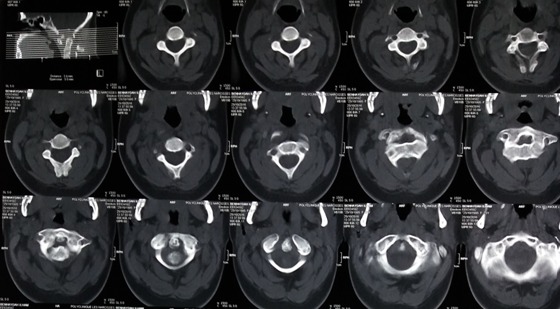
Cervical CT in axial section bone window showing C1-C2 dislocation with significant recoil of the odontoid process
